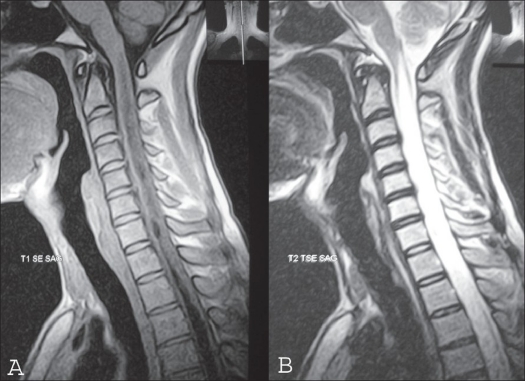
Mid sagittal T1-weighted image of MRI cervical spine (A) and T2 WI (B) shows syringomyelic cavity extending up to the medulla
